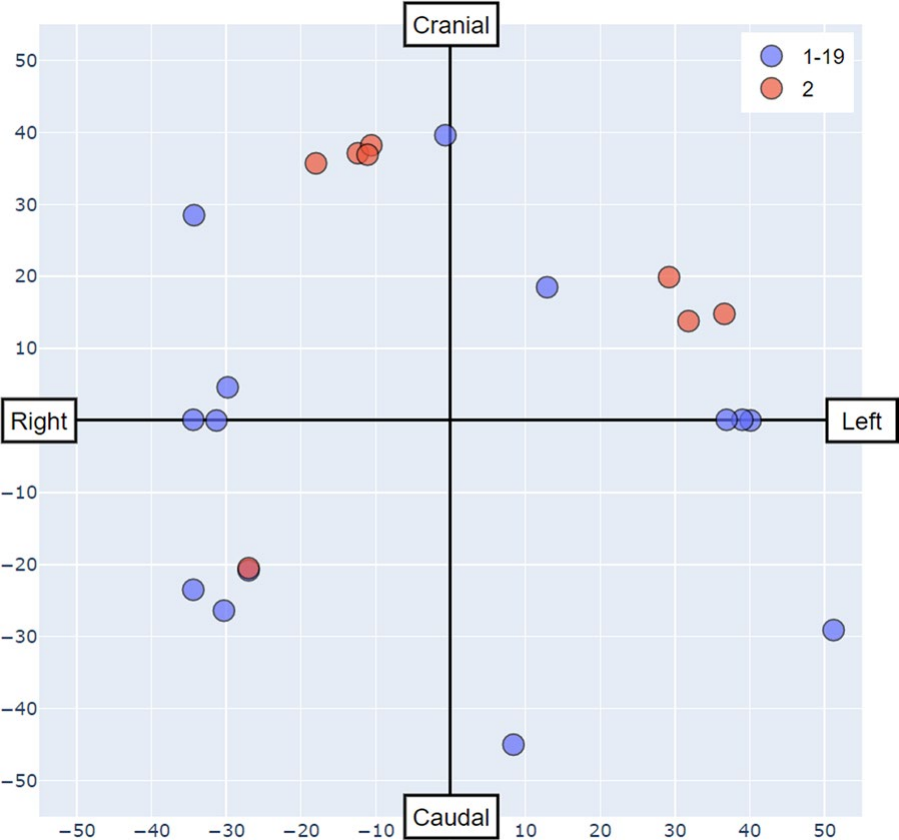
Image acquisition angles for Dataset 1-19 and Dataset 2. “Caudal” and “Cranial” refer to the caudal and cranial angulation of the X-ray
